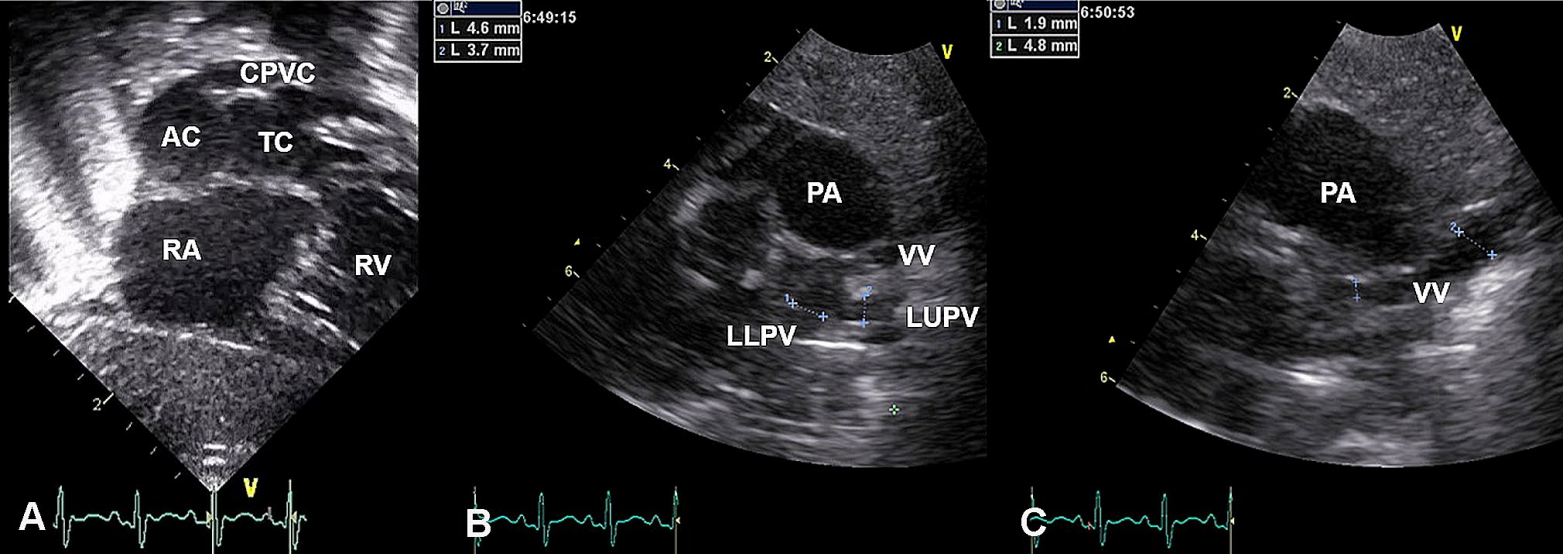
Preoperative transthoracic echocardiography. a The fibromuscular membrane dividing the left atrium and the common pulmonary venous collector (CPVC) connecting to the accessory left atrium (AC). b The left upper/lower pulmonary vein (LUPV/LLPV) draining into the vertical vein (VV). c The vertical vein compressed from the dilated pulmonary artery (PA). *RA* right atrium, *RV* right ventricle, *TC* true left atrial chamber
